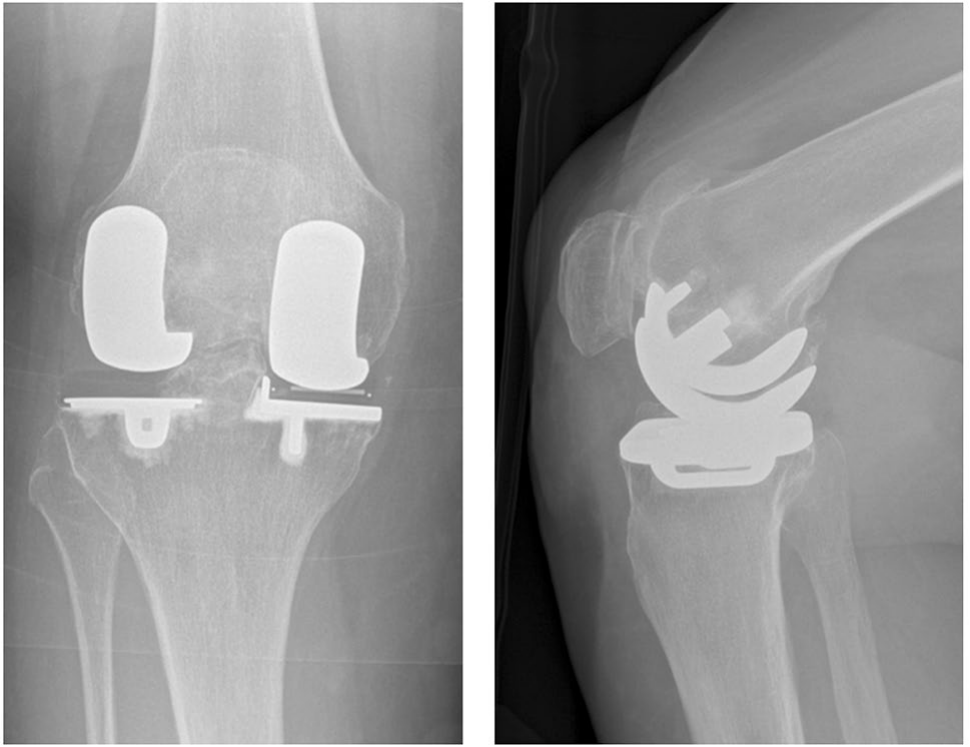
Postoperative AP-Xray (Left) and Sagittal-Xray (Right) of Fixed Lateral Oxford bi-unicompartmental knee replacement with well-aligned components (5-year OKS of 45 at last follow-up)
